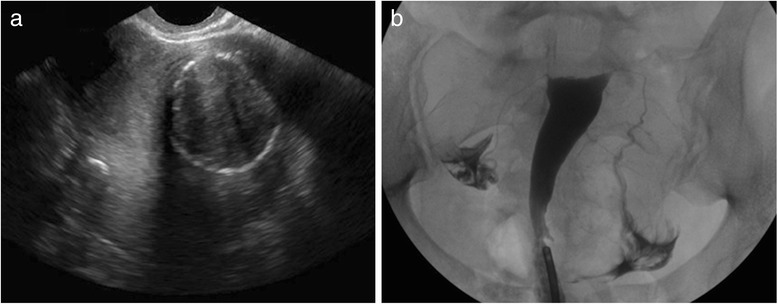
a 38-year-old G1P1 female with a history of infertility presenting for baseline assessment prior to initiation of ART. Coronal transvaginal sonographic image through the uterus demonstrates a 6.0 × 4.2 × 3.9 cm left sided mass with heterogenous echotexture and an echogenic rim, consistent with a large calcified intramural fibroid. b HSG demonstrates a normal endometrial cavity without filling defects to suggest fibroids as seen on TVS. The fallopian tubes are normal in caliber and demonstrate free intraperitoneal spill of contrast bilaterally
